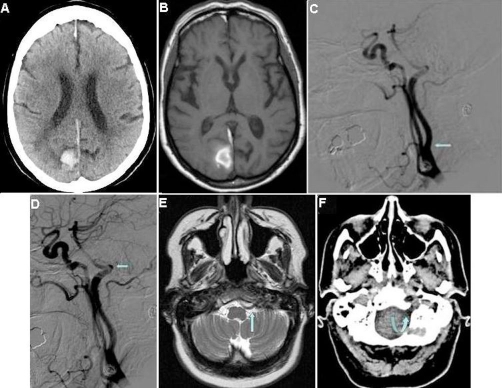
Bleeding in the right parieto-occipital lobe visualized on the non-contrast enhanced CT scan (A) and T1 weighted MRI (B). The lateral digital subtraction angiogram shows the hypoglossal artery originating from the carotid artery (C, arrow) and its conjunction with the basilar artery (D, arrow). The T2 weighted MR image (E, arrow) demonstrates the hypoglossal artery passing through the hypoglossal canal, which is enlarged as shown on the non-contrast enhanced-CT (F, curved-arrow).
